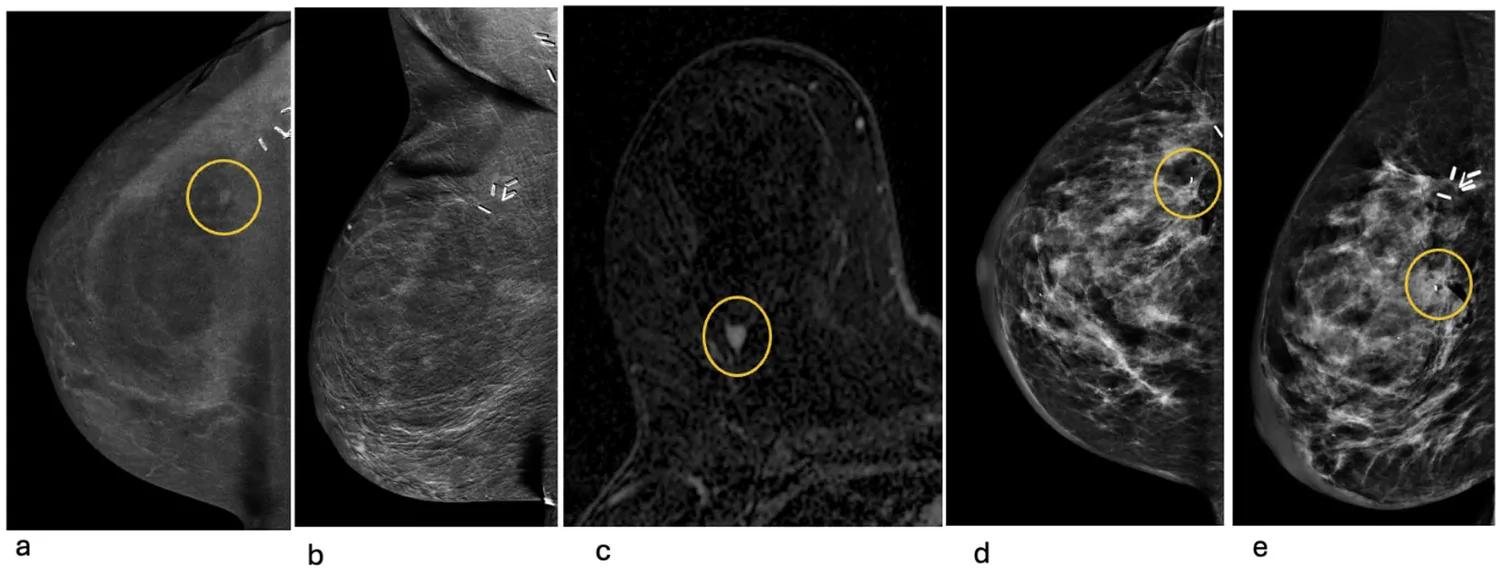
Low-conspicuity enhancing invasive lobular carcinoma. A 63-year-old woman with a history of right breast cancer and lumpectomy underwent routine screening CEM. On CEM, a low-conspicuity enhancing asymmetry is seen on the recombined images on the craniocaudal projection (a), without a clear, definite correlate on the mediolateral projection (b) or on the low-energy images (not pictured). Subsequent contrast-enhanced MRI (c axial post-contrast subtraction sequence) demonstrated a corresponding enhancing mass. MRI-guided biopsy was performed, yielding invasive lobular carcinoma. Post-procedure full-field digital mammogram (d craniocaudal projection and e lateral projection) demonstrated the biopsy marker in the location of the originally identified enhancing asymmetry
